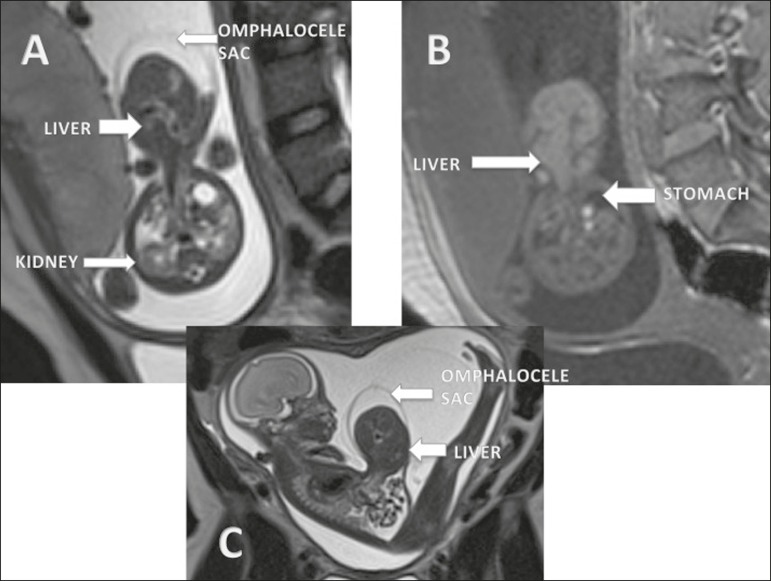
Omphalocele in a fetus at 22 weeks. A: Axial T2-weighted
